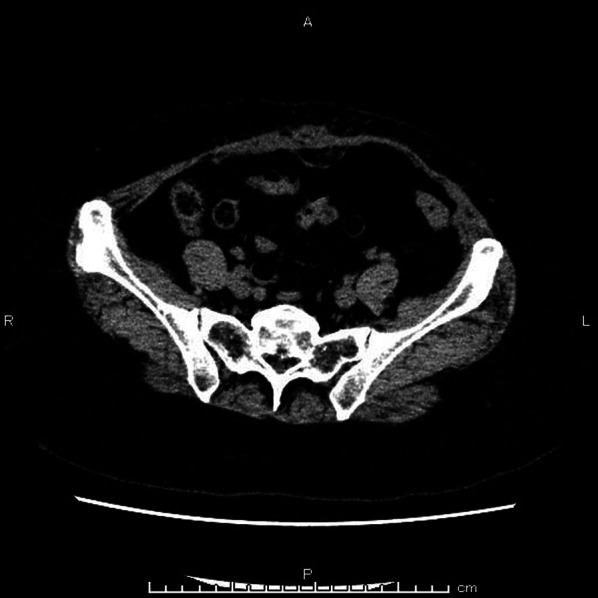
Postoperative abdominal computed tomography. Abdominal computed tomography revealed no abdominal bulge recurrence occurred after 2 months of follow-up
